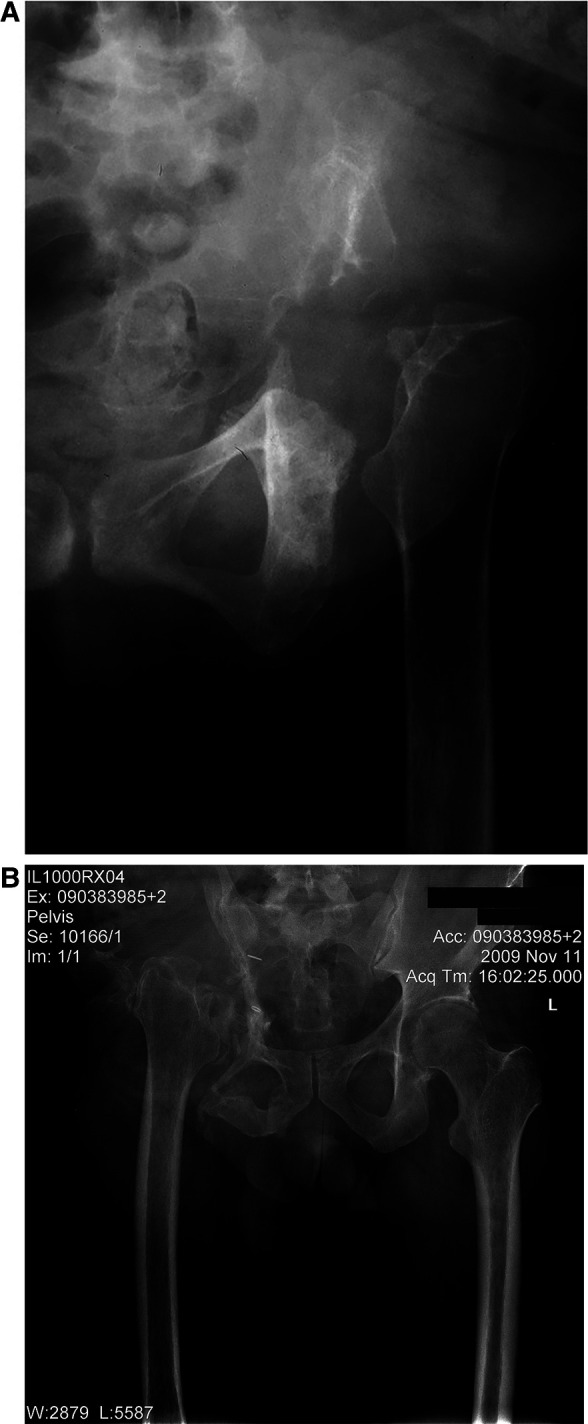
(A,B) plain anterior-posterior (AP) radiograph of the hemipelvis showing proximal migration of the operated extremity after bone tumor resection following six weeks of weight bearing. (A) shows the left hemipelvis, and (B) the right hemipelvis.
